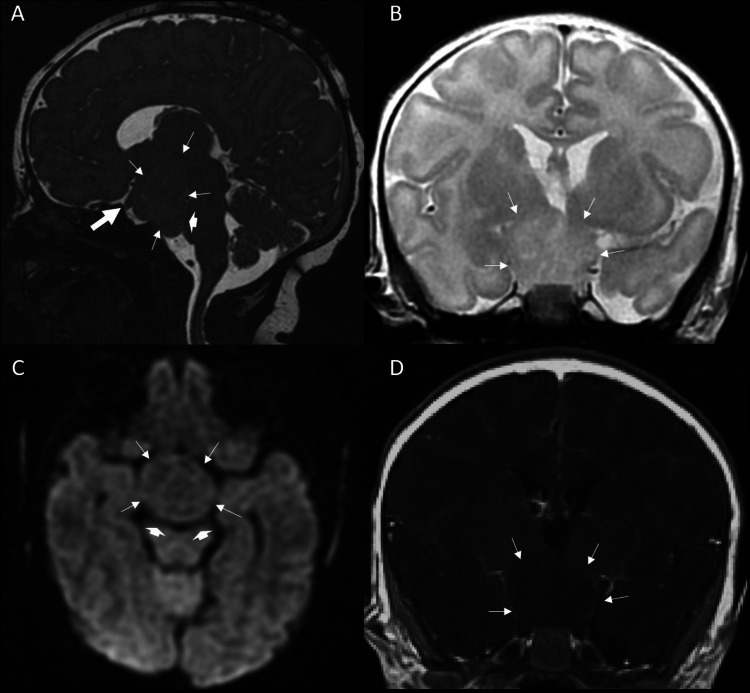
First week of life brain MRI A: Sagittal 3DT2, B: Coronal T2W, C: Axial DWI, and D: Coronal postcontrast T1W of the brain MRI in the first week of life demonstrates large suprasellar mass (outlined by the arrows) compatible with hamartoma. The signal characteristics within the hamartoma on T1W (D) and T2W images (A and B) are identical to the intensities of the normal grey matter and the non-myelinated white matter. The lesion involves the hypothalamus and fills the suprasellar, perimesensephalic and upper prepontine cisterns. There is a mild mass effect upon the optic chiasm which is anteriorly and superiorly displaced (thick arrow on A). There is also a mass effect upon the midbrain and pons, causing flattening of the cerebral peduncles (arrowheads on C) and ventral pons (arrowhead on A). Postcontrast imaging demonstrates no abnormal enhancement (D). DWI characteristics are also similar to the brain without evidence of hypercellular process (C). MRI = Magnetic resonance imaging, 3DT2 = 3 dimensional T2, T2W = T2 weighted, DWI = Diffusion weighted imaging, T1W = T1 weighted
